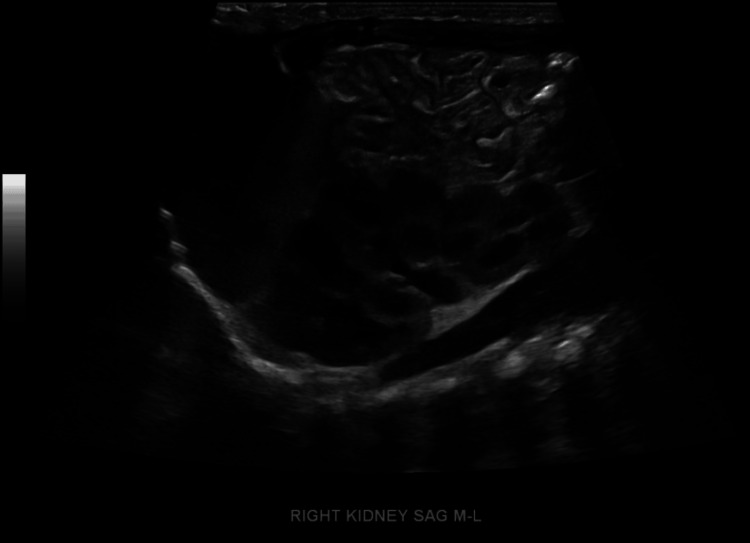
Complete Renal Ultrasound of Right Kidney Ultrasound showing mild right-sided hydronephrosis.
